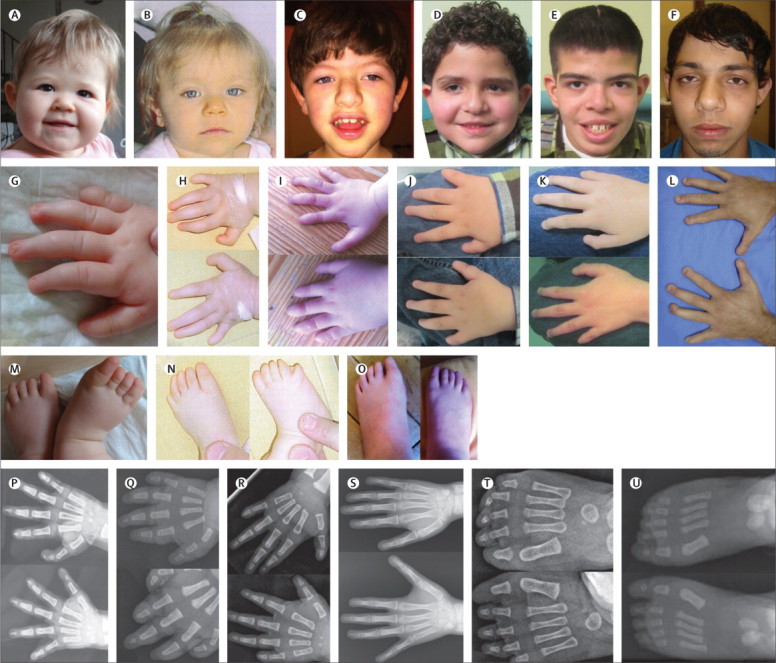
Physical features of participants with *TBC1D24* mutations (A–F) Some individuals have a wide base of the nose and bulbous end of the nose (individual numbers from tables corresponding to each panel: A=3, B=6, C=5a, D=2b, E=2a, F=7). (G–L) Hands in individuals with *TBC1D24* mutations have triphalangeal thumbs, brachydactyly, short terminal phalanges, and hypoplasia or aplasia of the nails (G=3, H=6, I=5a, J=2b, K=2a, I=7). (M–O) Feet with short terminal phalanges and hypoplasia or aplasia of the nails (M=3, N=6, O=5a). (P–S) Radiographs of the hands in individuals with *TBC1D24* mutations. Note the triphalangeal thumbs and the short terminal phalanges (P=4, Q=5a, R=6, S=1). (T,U) Radiographs of the feet in individuals with *TBC1D24* mutations, showing short terminal phalanges (T=6, U=5a).
